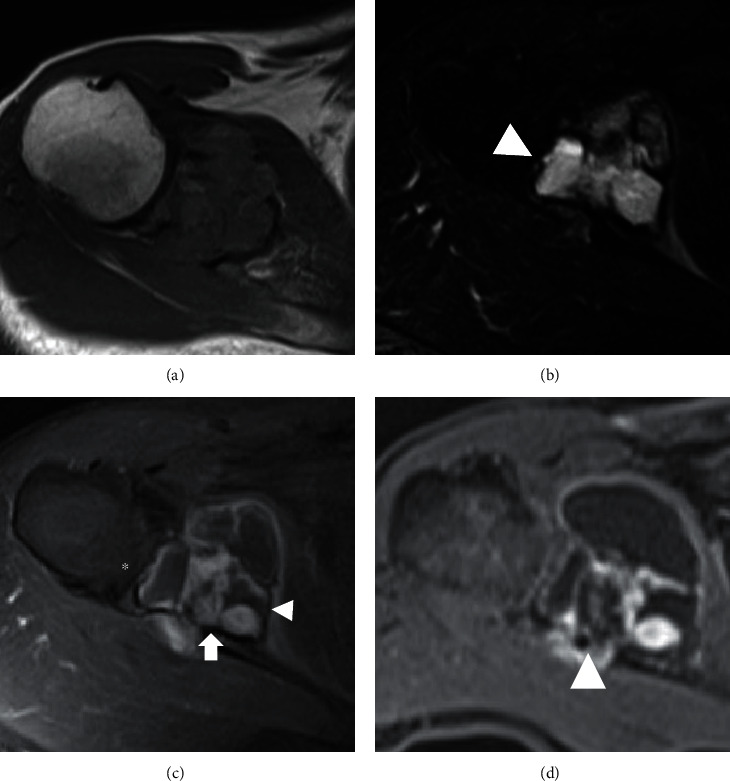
Magnetic resonance imaging at first presentation (a)–(c), and postchemotherapy (d). The lobulated tumor is located at the scapula and is seen invading into the anterior and posterior soft tissue. (a) Axial T1-weighted image shows slightly high signal intensity. (b) Axial T2-weighted image depicts high signal intensity-multilocular mass with low signal intensity rim, and a part of the nodule shows fluid-fluid level (white arrowhead). (c) Axial Gd enhanced T1-weighted image represents thin enhancement surrounding low-intensity rim and enhanced solid nodule (arrow). Partial invasion into soft tissue is observed (asterisk). (d) Axial Gd enhanced T1-weighted image after chemotherapy shows cystic change of solid nodule compared with prechemotherapy image in (c) panel.
